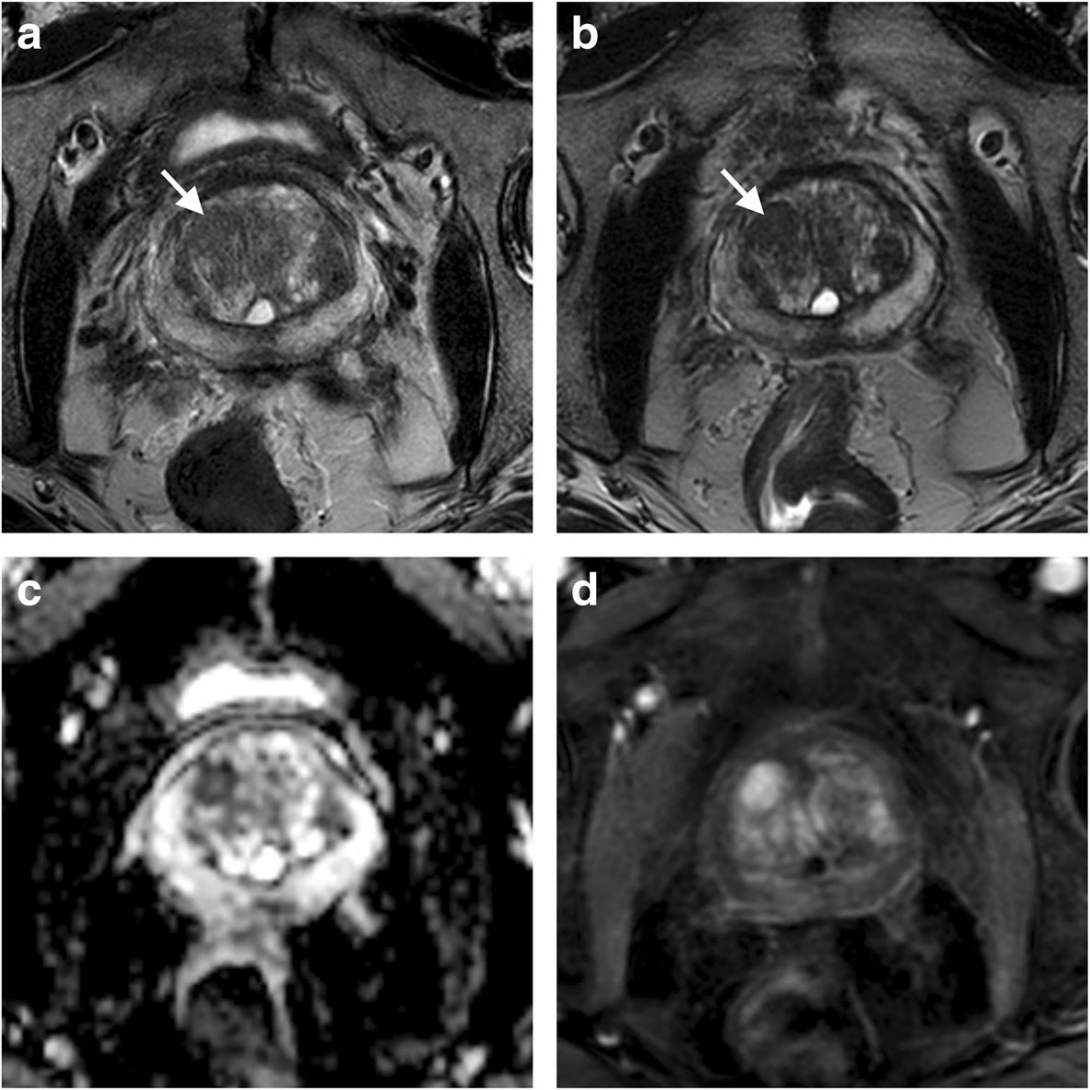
Three-dimensional (3D) T2-weighted imaging in a biopsy-naïve 69-year-old patient with elevated PSA (5.39 ng/mL) undergoing mpMRI for the purpose of targeted biopsy. The examination showed a nodule in the right TZ showing moderate hypointensity on transverse two-dimensional T2-weighted imaging and somewhat ill-defined margins (arrow in a). 3D T2-weighted imaging better delineated the nodule margins as a “charcoal” peripheral rim (arrow in b) by reducing image blurring, thus contributing to characterise it as a benign prostate hyperthropy (BPH) fibrostromal nodule. The nodule showed restricted diffusion (hypointensity on the ADC map in c) and intense, early contrast enhancement on transverse fat-saturated T1-weighted imaging (d)
